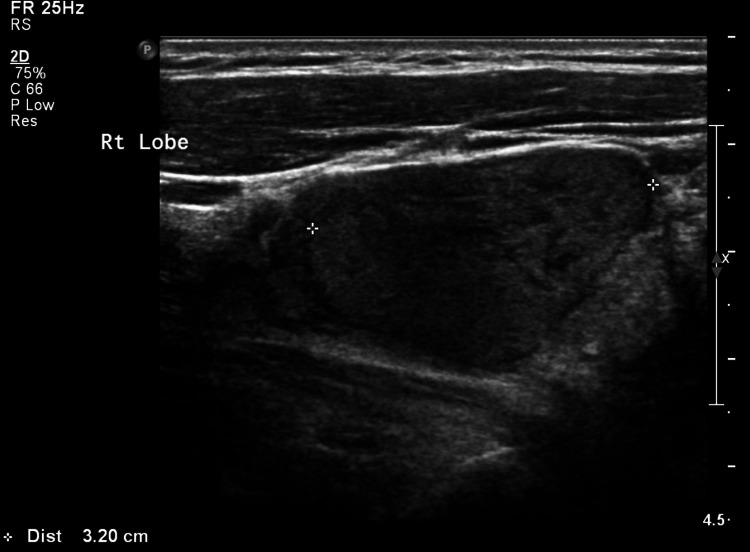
Largest thyroid nodule. Right thyroid ultrasound image showing the largest nodule measuring 3.2 cm.
